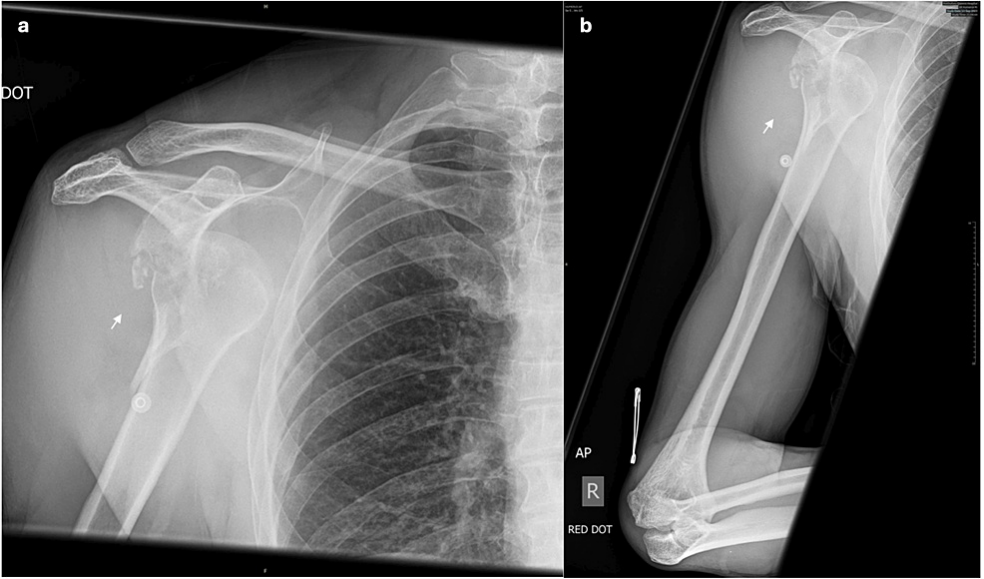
Pre-operative radiographs. a. Anterior-posterior X-ray. b. Humerus X-ray. Figures 6a-6b show a fracture dislocation of the glenohumeral joint with evidence of a comminution and dorsal displacement of fracture fragments (white arrows).
